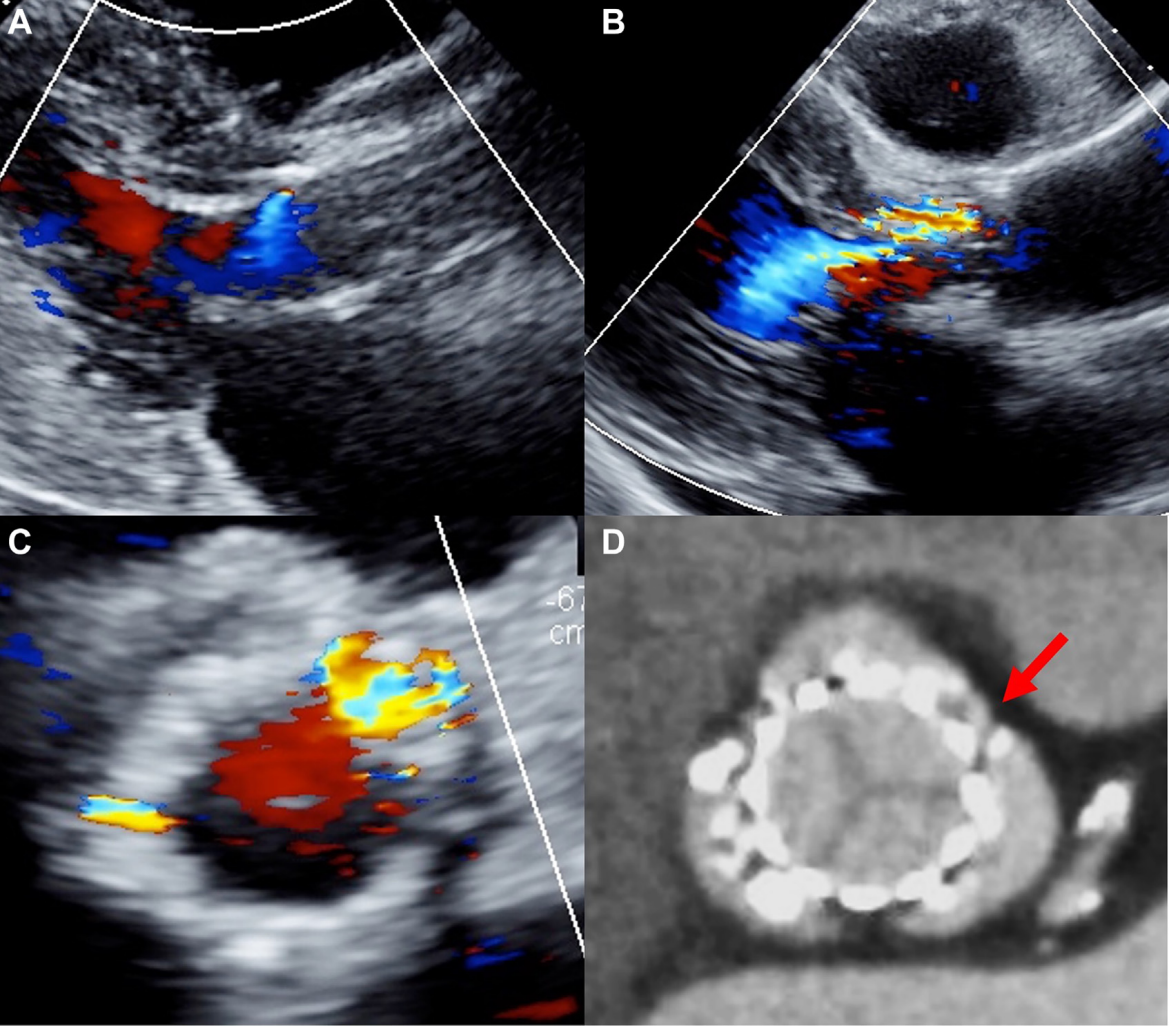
Progression of Pravalvular Leak (A) Transthoracic echocardiography at discharge. Paravalvular leak was mild. (B and C) Transthoracic echocardiography at 1 month (B: long-axis view, C: short-axis view). Paravalvular leak progressed to moderate. Main paravalvular leak originated from 1 o’clock position between left and right coronary cusps. (D) Computed tomography showed the gap between left and right coronary cusps (red arrow).
